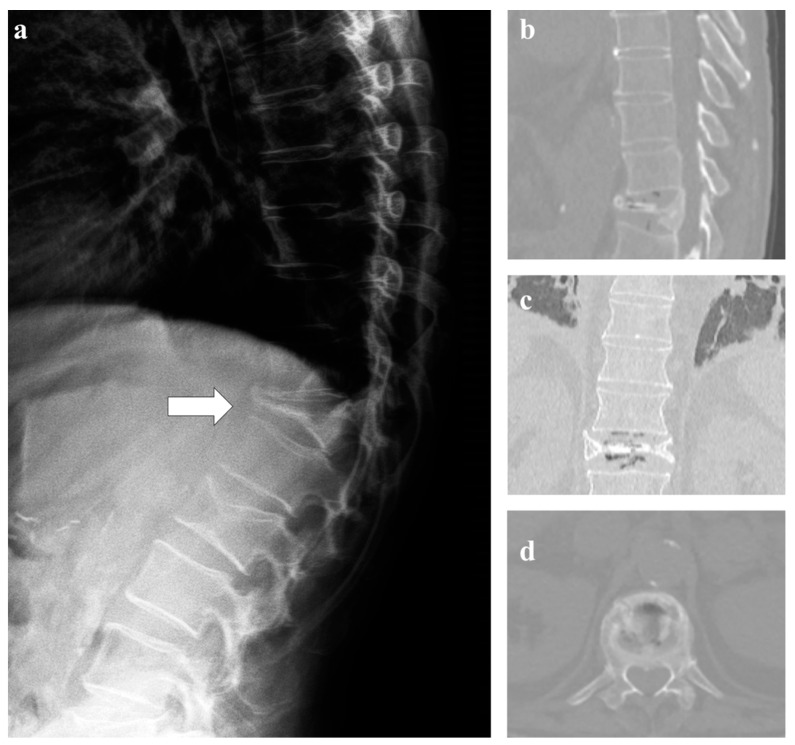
A 62-year-old male was admitted with severe backache after a long history of prednisolone therapy for rheumatoid arthritis. (a) A diagnosis of Kummel disease has been performed after a progressive collapse of the vertebra. Sagittal (b), coronal (c) and axial (d) CT scan shows intra-vertebral “vacuum” associated with air in the adjacent disc spaces, signs of symptomatic vertebra plana.
